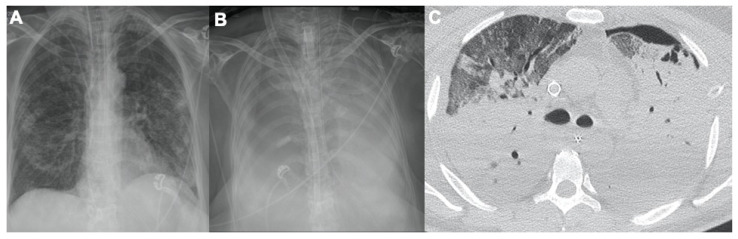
Evolution of COVID-19 pneumonia in the same patient (52-year-old male). (A) Day 9 from infection: peripheral opacities, 2 central venous catheters, endotracheal tube and nasogastric tube. (B) Day 14 from infection: both lungs are fully consolidated with bilateral pleural effusion, V-V ECMO, and tracheostomy. (C) CT at day 20, ARDS with left pneumothorax and chest tube positioned.
